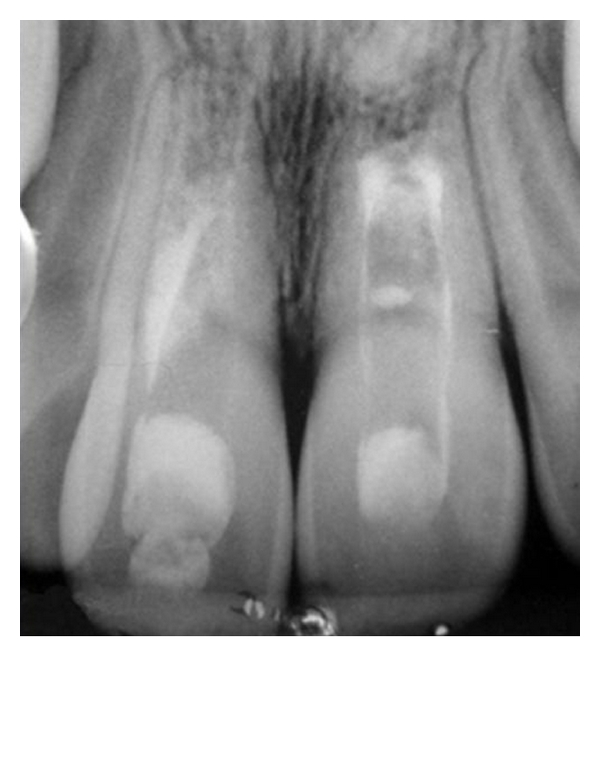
Twenty-month posttreatment radiograph (Sep. 2008).
